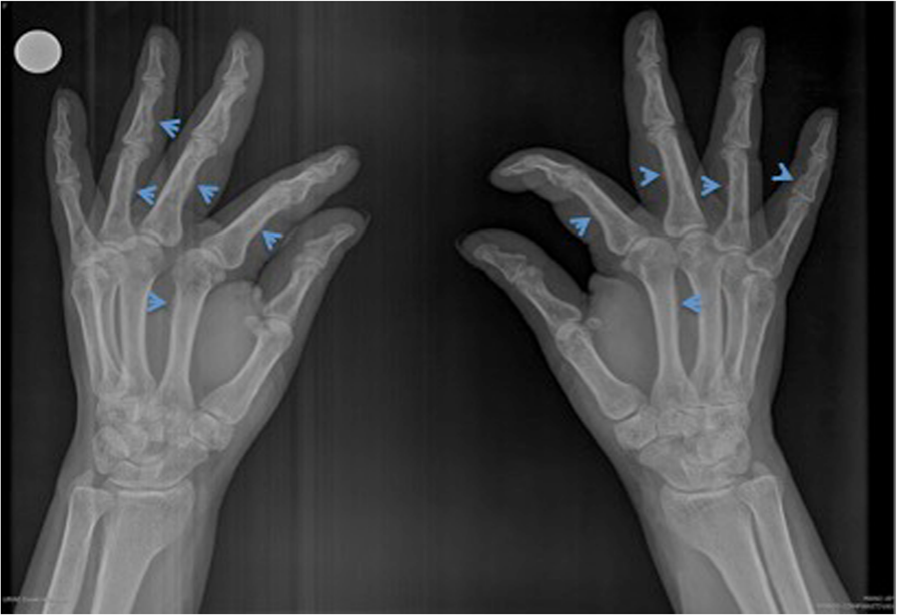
Radiography of our patient’s hands. Radiography showed periostitis of phalangeal and metacarpal bones (*arrowheads*) and joint space narrowing
